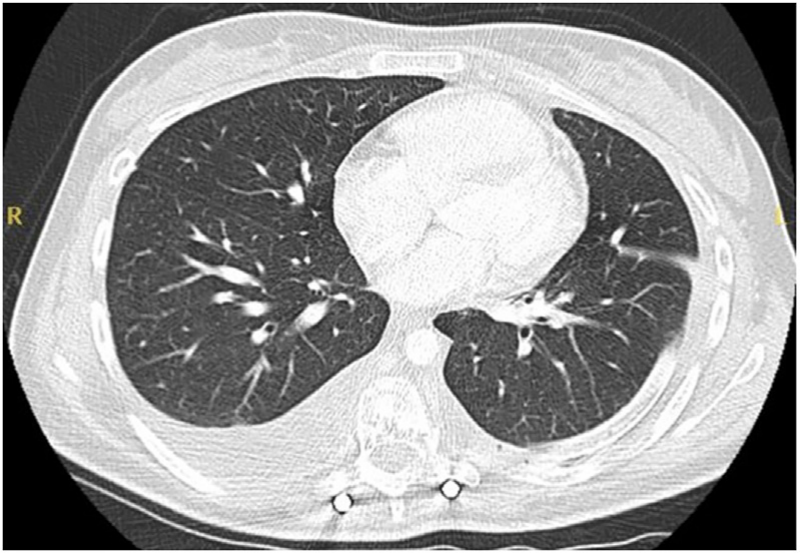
Computed tomography of the thorax demonstrating resolution of the left pleural effusion with outline of chest drain in situ.
